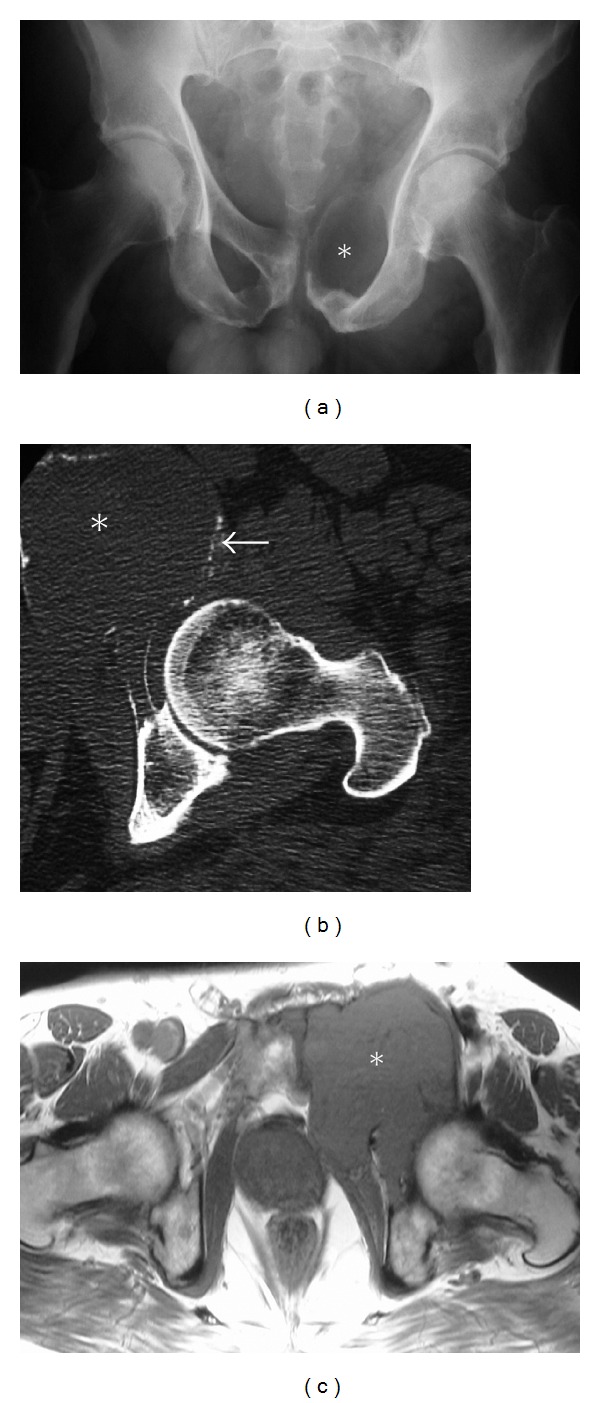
Plasmacytoma. A 68-year-old male with plasmacytoma. Radiographs (a) of the pelvis showing expansion of the left obturator foramen and an expansile osteolytic lesion involving the superior pubic ramus (asterisk). Axial CT (b) depicts the large lytic mass expanding the superior pubic rami (asterisk). A thin mineralized periphery in keeping with expanded cortex is appreciated on CT (arrow). Axial T1 MR (c) demonstrates the expansile mass (asterisk) displacing the left femoral vessels, indenting the left anterolateral aspect of the prostate and invading the adjacent obturator internus muscle.
